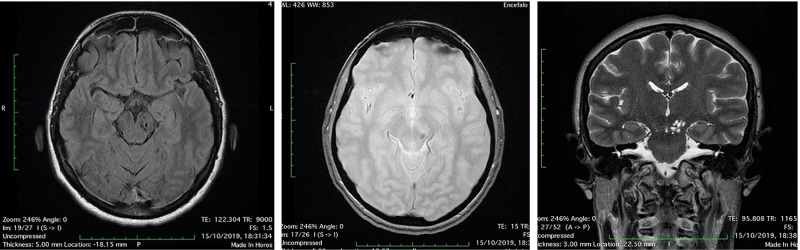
Head MRI scan “after four months”: the cystic lesion was reduced, with punctiform hemosiderin sign (A: FLAIR axial image; B: T2 gradient echo axial image; C: T2 coronal image).
